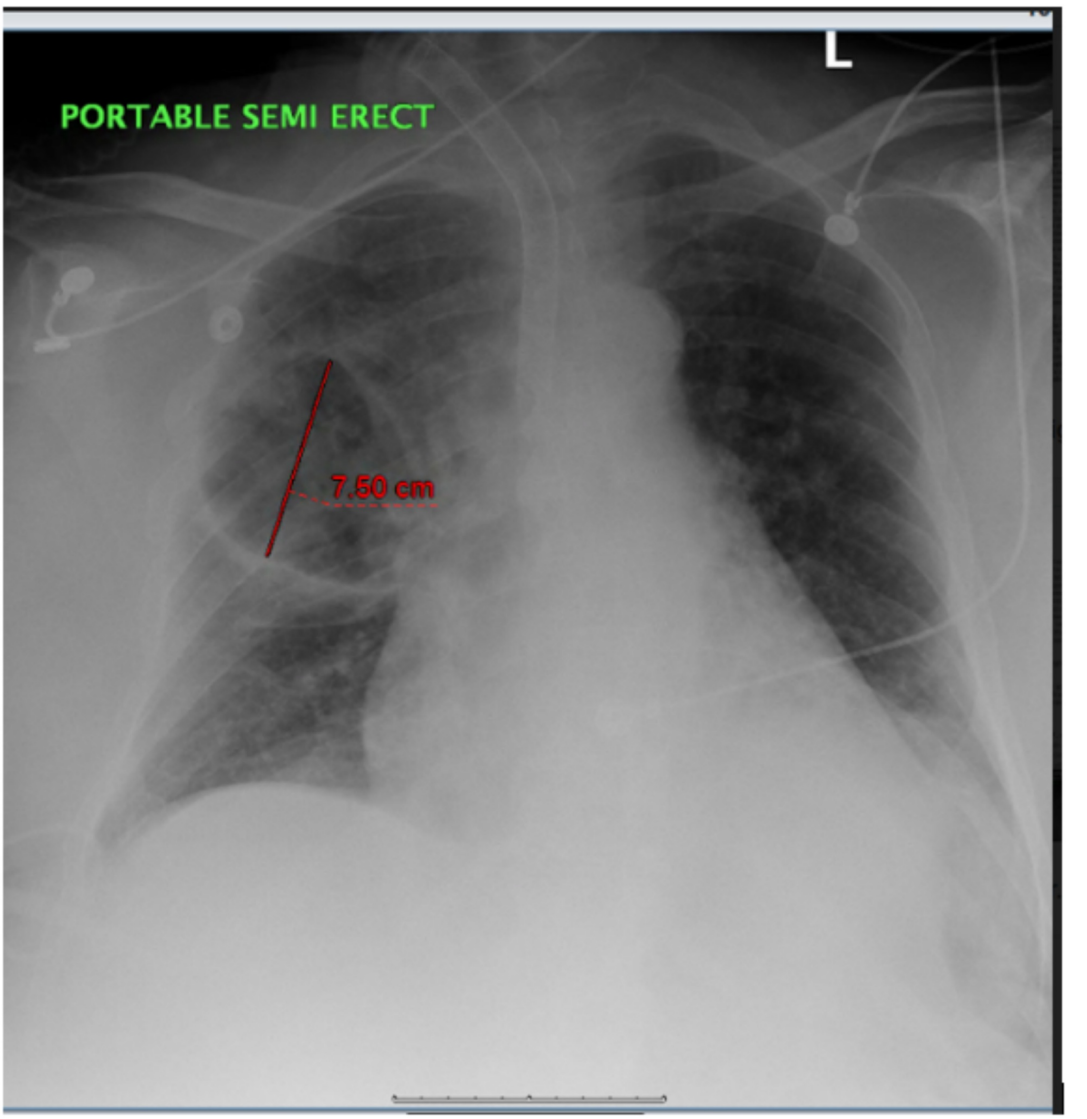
Chest X-ray: There is patchy infiltrate versus atelectasis at the left lung base. There are patchy infiltrates in the right upper lobe. There is also new thick-walled cystic airspace in the right upper lobe since the previous study.
